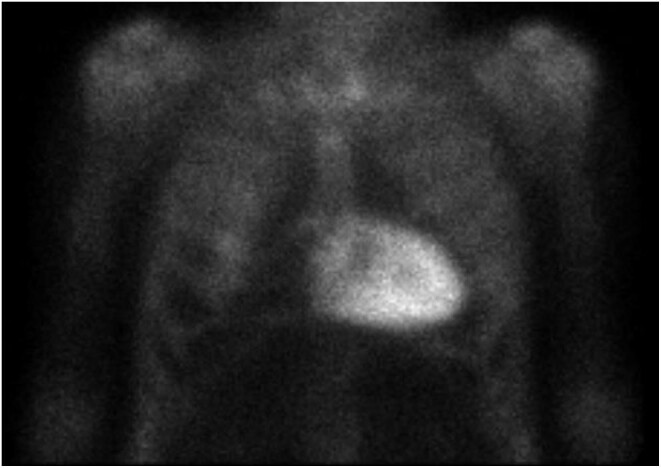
99mTc-DPD scintigraphy showing high- grade cardiac uptake.
